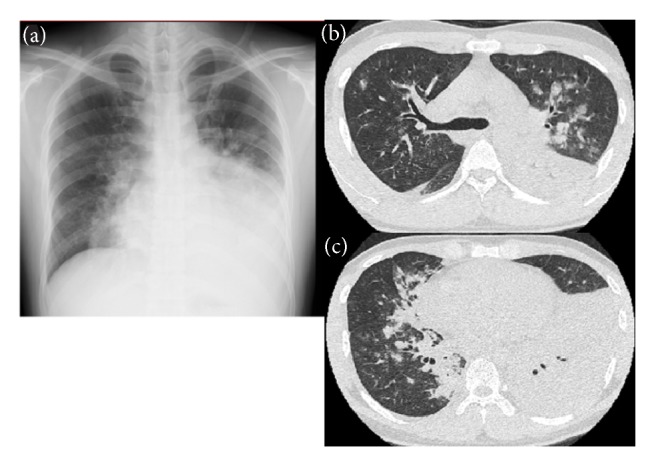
Chest radiography and chest computed tomography findings. (a) Chest radiography taken at the time of hospitalization, showing an infiltrating shadow in left middle lung field and right lower lung field. (b)-(c) Computed tomography scan taken at the time of hospitalization, showing lobar pneumonia in the lower left lobe and infiltrating shadow in the left whole lung lobe and the lower right lobe.
